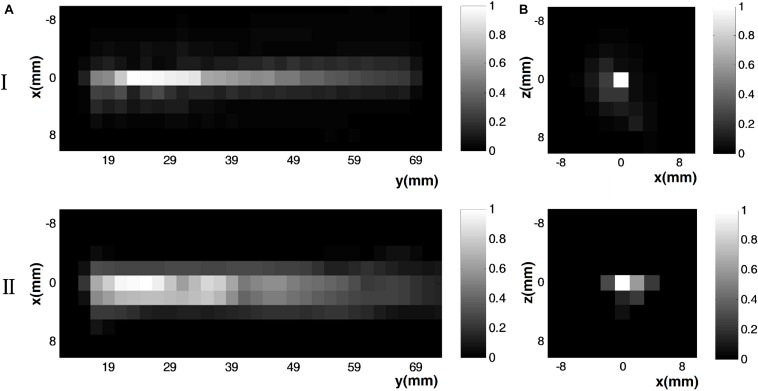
Measurement results of (I) the ultrasound pressure field distribution and (II) the electrical field intensity distribution at (A) the *x*–*y* section (*y* = 0 mm) and (B)
